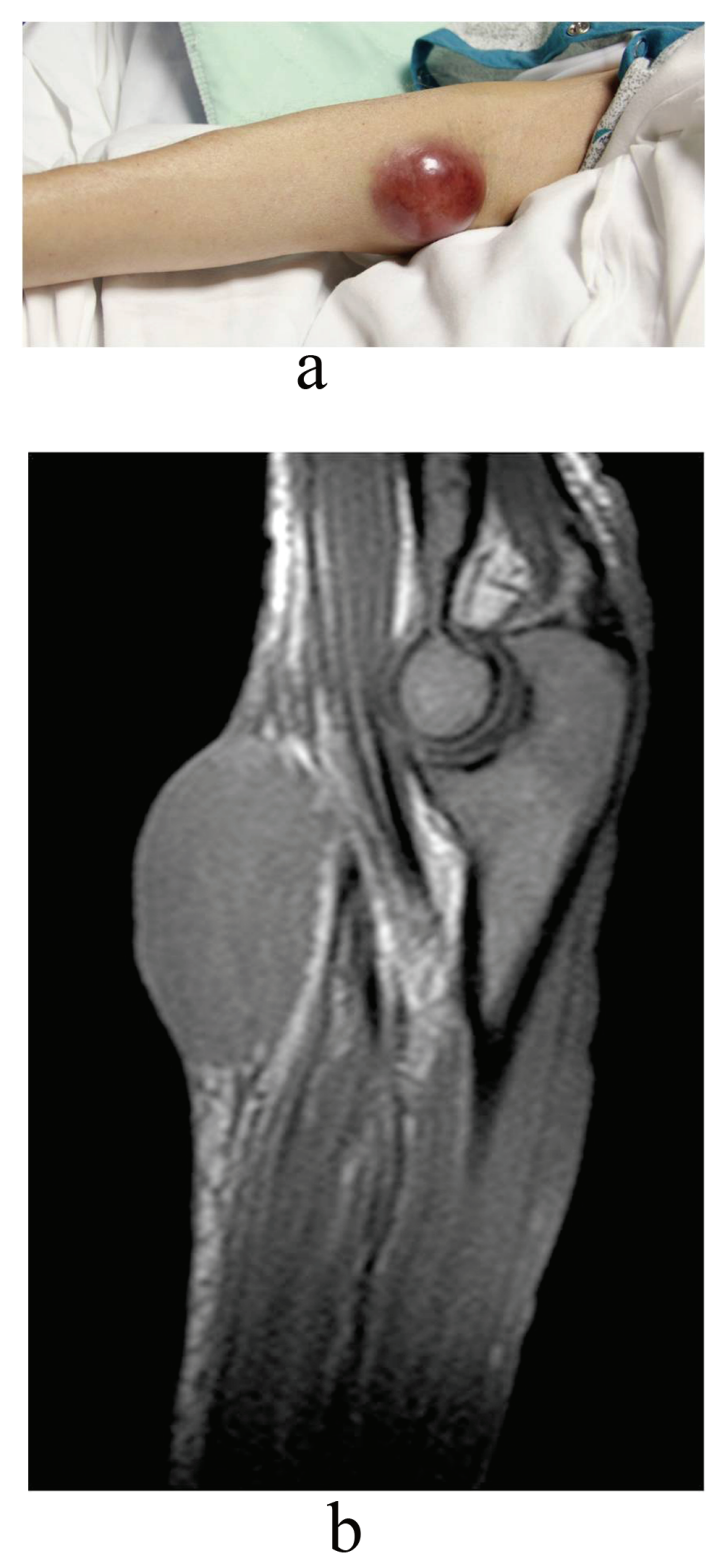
a). Right elbow suggestive of possible “hematoma” and b) MRI of right elbow suggested deposit of lymphoma.
